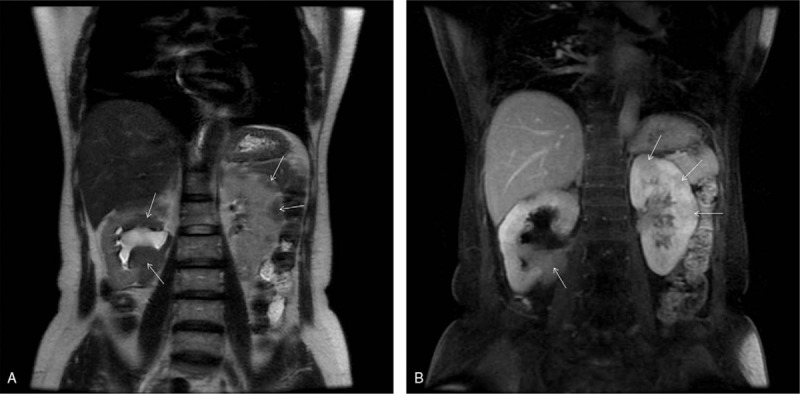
IgG4-RKD presentation on MRI, displayed as coronal T2WI (A) and postcontrast T1WI (B). The right pelvic mass and bilateral multiple renal parenchyma lesions were hypointense on T2WI compared with the normal renal parenchyma, with mild enhancement on contrast T1WI (arrows). IgG4-RKD = IgG4-related kidney disease, MRI = magnetic resonance imaging, T1WI = T1-weighted imaging, T2WI = T2-weighted imaging.
